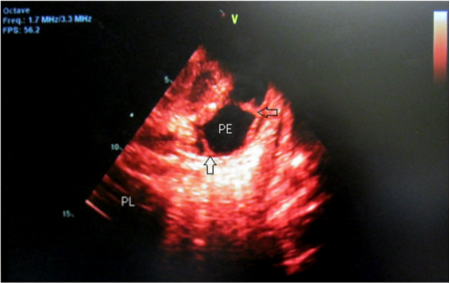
Two-dimensional transthoracic echocardiography was also remarkable for mobile fibrinous strands (arrows) between the visceral and parietal pericardium, as well as confirmation of a left-sided pleural effusion (PL).
